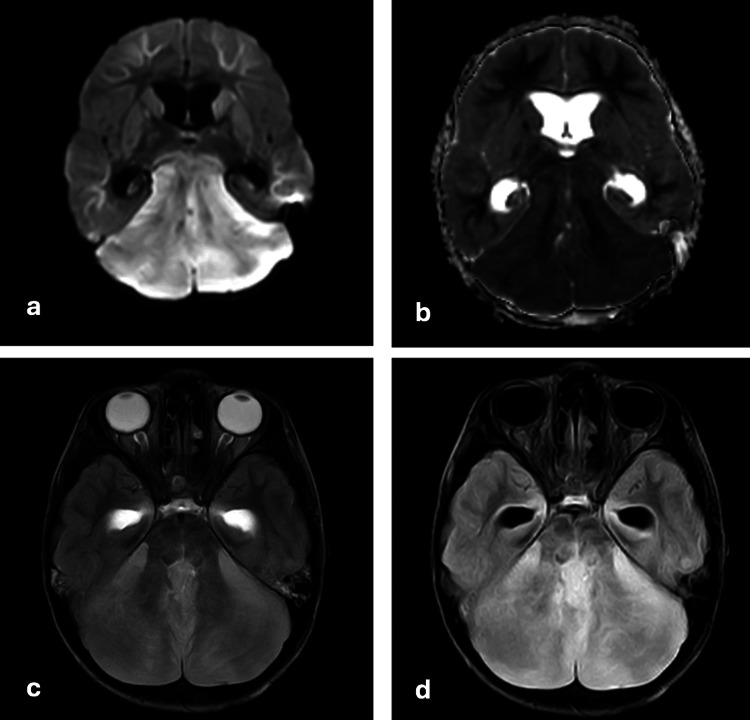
MRI brain axial sections depicting bilateral cerebellar hemispheres showing restricted diffusion on (a) DWI sequence with corresponding low signal intensity, (b) ADC sequence appearing homogenously hyperintense, (c) T2 showing evidence of increased T2 signal intensity around the bilateral optic nerves, and (d) FLAIR sequences MRI: magnetic resonance imaging; DWI: diffusion-weighted imaging; ADC: apparent diffusion coefficient; FLAIR: fluid-attenuated inversion recovery
